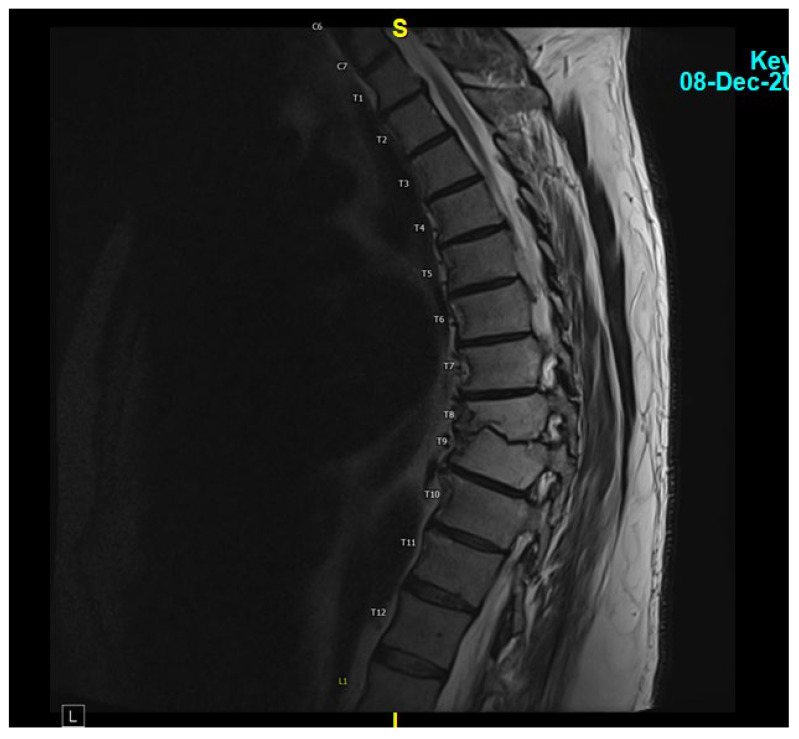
MRI thoracic spine with and without IV contrast showing a completely resolved T8-T9 disc space infection.
